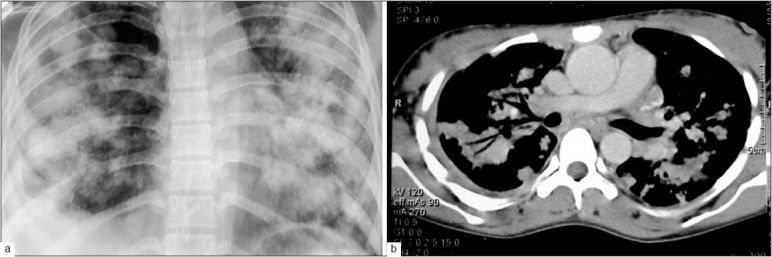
a) Chest radiograph PA view showing bilateral diffuse alveolar opacities having irregular fluffy margins, showing a pattern of coalescence and presentation with butterfly distribution. The blunting of right CP angle is suggestive of pleural effusion; b) CT thorax showing the diffuse alveolar opacities and presence of right pleural effusion
